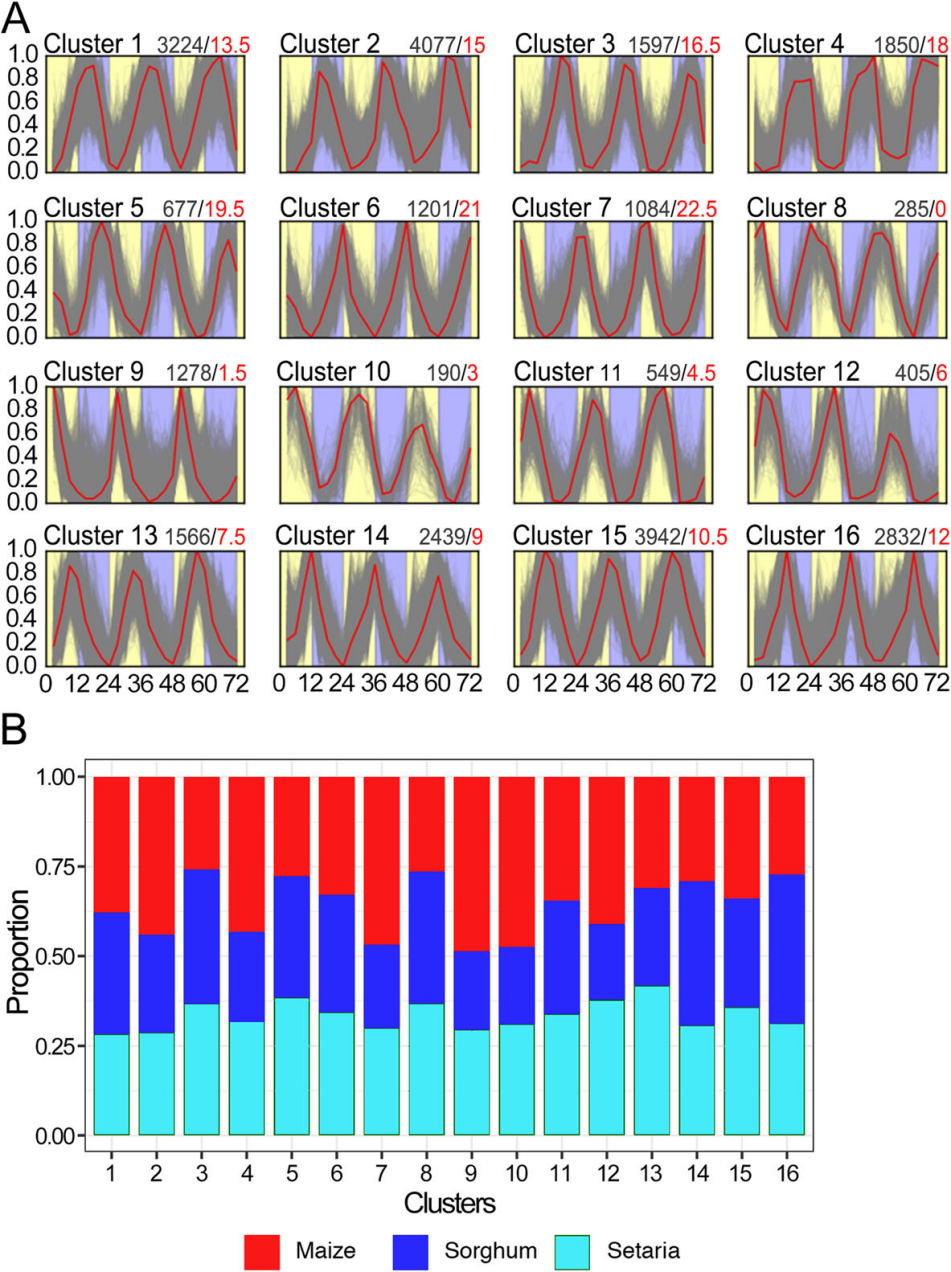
Diurnal co-expression clusters for orthologous syntenic sorghum, maize, and foxtail millet genes. a Normalized gene expression values show the diurnal expression pattern in 16 clusters derived from K-means clustering. b Proportion of genes from maize (red), sorghum (dark blue), and foxtail millet (light blue) in each of the 16 clusters. In (a), x-axis shows the time points and y-axis shows the 0–1 scaled FPKM values for each gene. Yellow and blue areas represent light and dark periods, respectively. Heavy red line is the median expression pattern of genes in that cluster and fine grey lines are individual genes. The total number of genes and median phase (in CT) of each cluster are show at the top of each panel as: total gene number/median phase (in red text)
